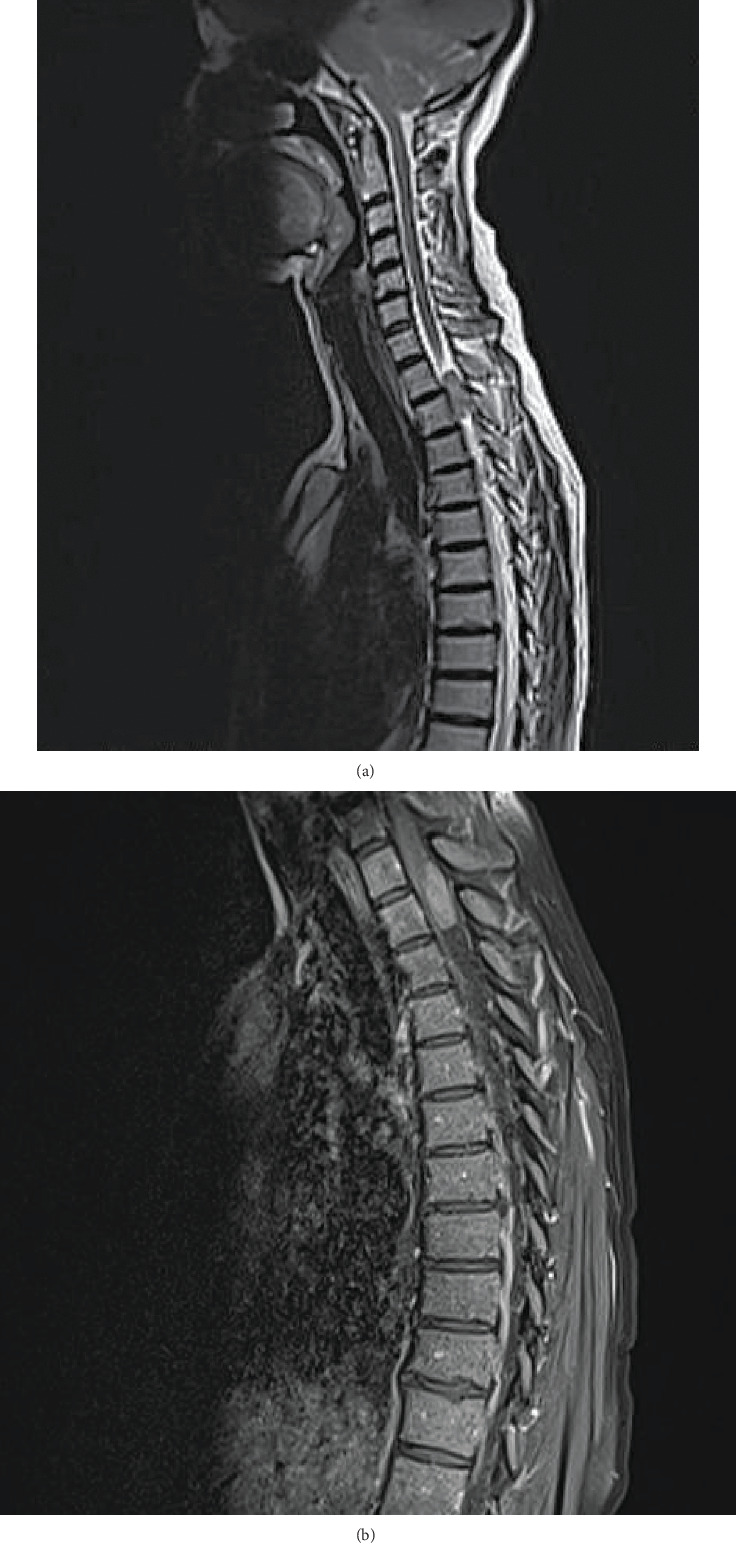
Contrast-enhanced magnetic resonance imaging of the thoracic spine before surgery. (a) Sagittal T_2_-weighted sequence demonstrates a small mass in the upper thoracic spine with primarily high T_2_ signal. (b) Upon contrast application T_1_-weighted sequence demonstrates strong, quite homogeneous mass enhancement.
